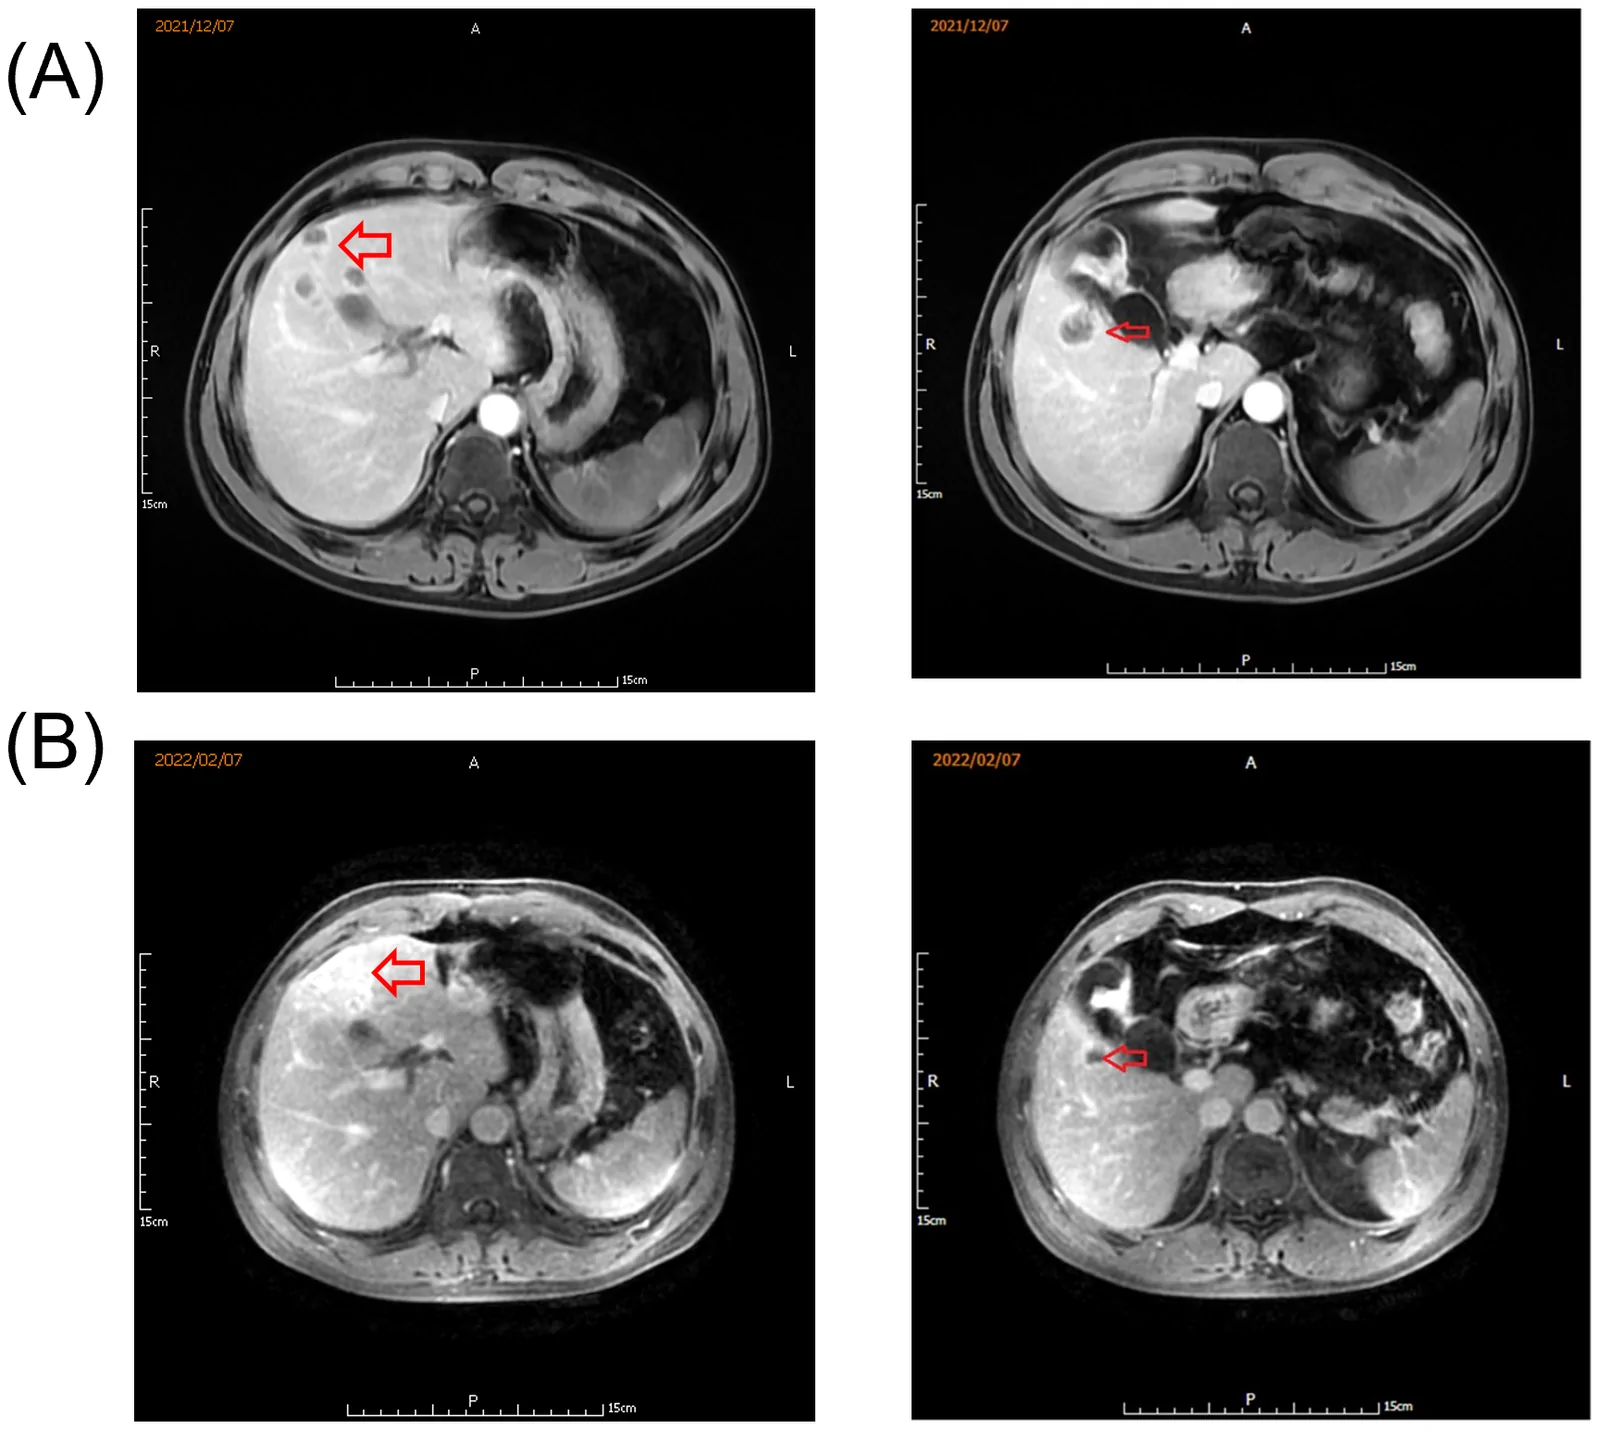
Serial abdominal MRI images of hepatic metastasis before and after T-DM1 plus sintilimab therapy. (A) Abdominal MRI obtained after two cycles of gemcitabine, oxaliplatin, lenvatinib and sintilimab therapy; no obvious reduction of the hepatic lesion was observed. (B) Abdominal MRI after two cycles of trastuzumab emtansine combined with sintilimab treatment. Obvious qualitative shrinkage of the target lesion can be appreciated. Precise quantitative assessment of tumour size was not feasible due to inconsistent slice positioning and respiratory phases across scans.
